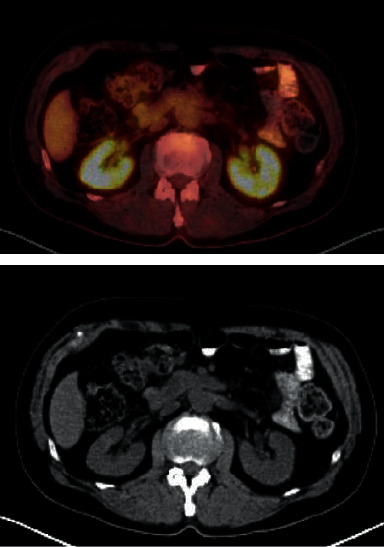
Diffuse symmetric fluorodeoxyglucose (FDG) uptake within the bilateral renal parenchyma on positron emission tomography-computed tomography (PET-CT) scan.
